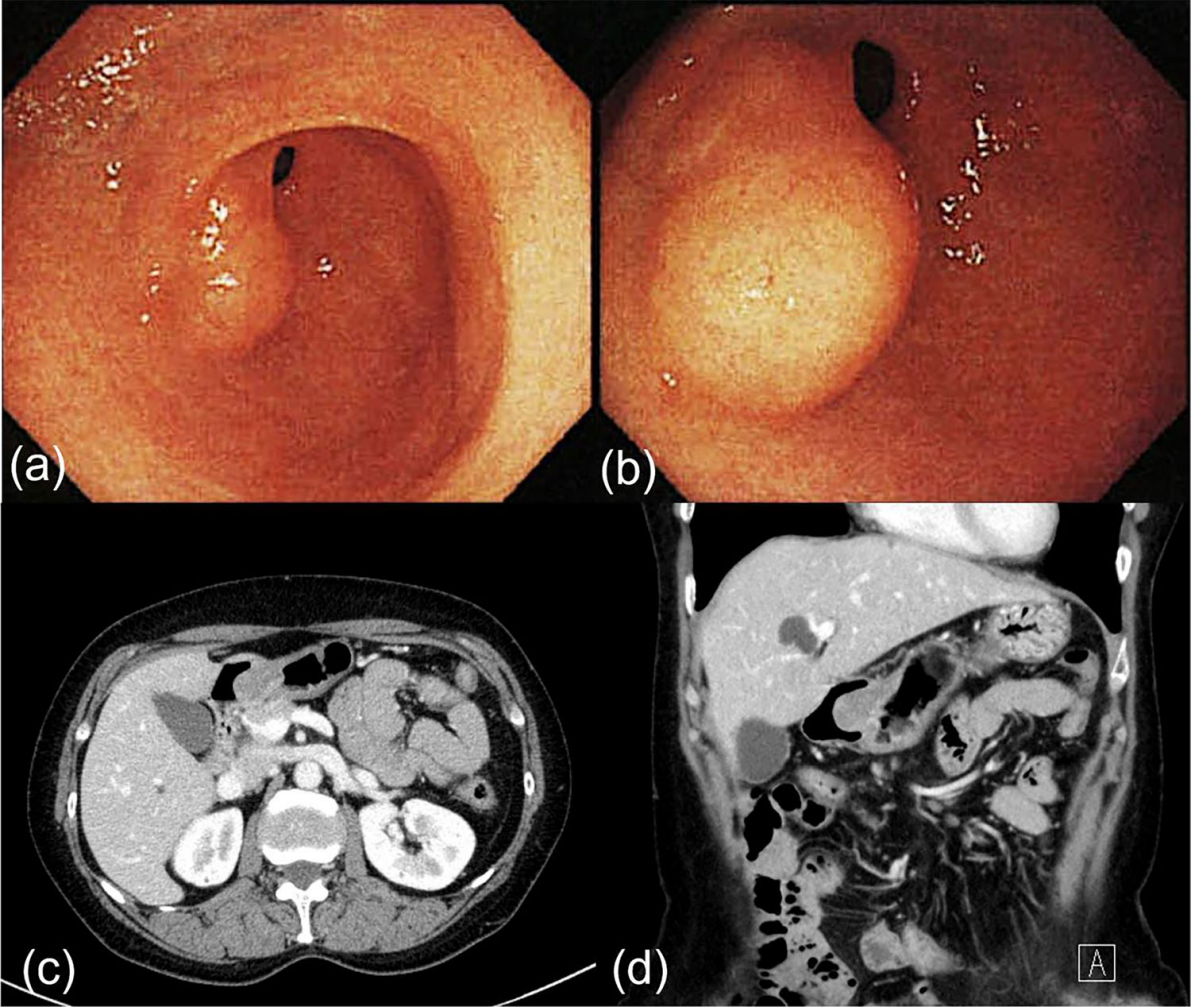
(a, b) Upper gastrointestinal endoscopy reveals a gastric submucosal tumor with a smooth surface at the gastric antrum. (c, d) Contrast‐enhanced computed tomography demonstrates a rim‐enhanced mass (20 mm in diameter) protruding into the lumen of the gastric antrum.
